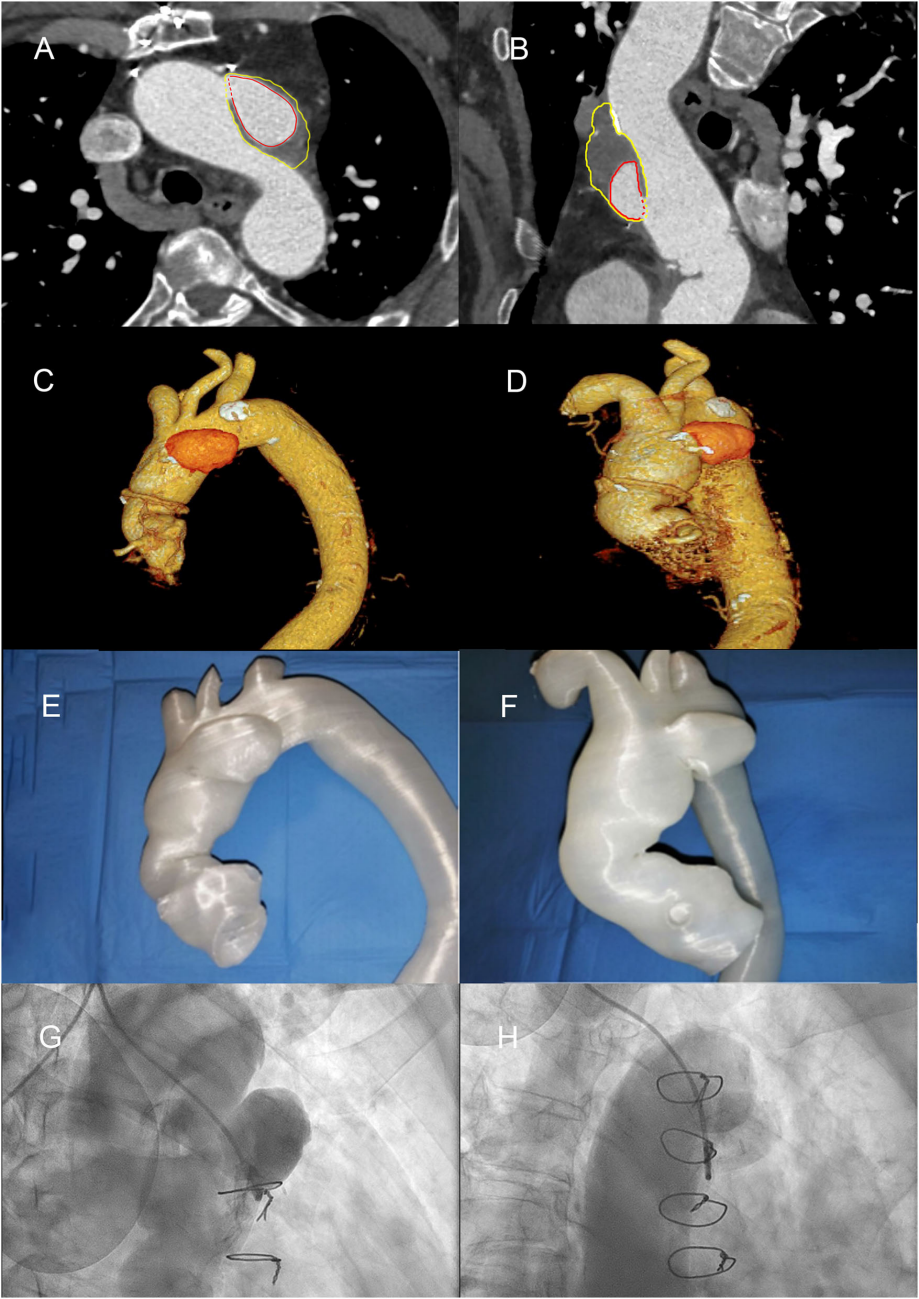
Diagnostic angiography, computed tomography scans, and 3D printed thoracic aorta model, demonstrating aortic root, and aortic arch pseudoaneurysm (AAP). (A) Computed tomography (CT) angio-study, axial image, showing pre-treatment of AAP. The red line highlights the perfused part of the AAP; the yellow line highlights the thrombosed part. (B) CT angio-study, curved multiplanar reconstruction (MPR) of AAP. (C,D) Volume rendering (VR) reconstructions of ascending aorta. (E,F) 3D print of the thoracic aorta and AAP. (G) Right anterior oblique (RAO) cranial angiographic image of AAP. (H) RAO caudal angiographic image of AAP.
